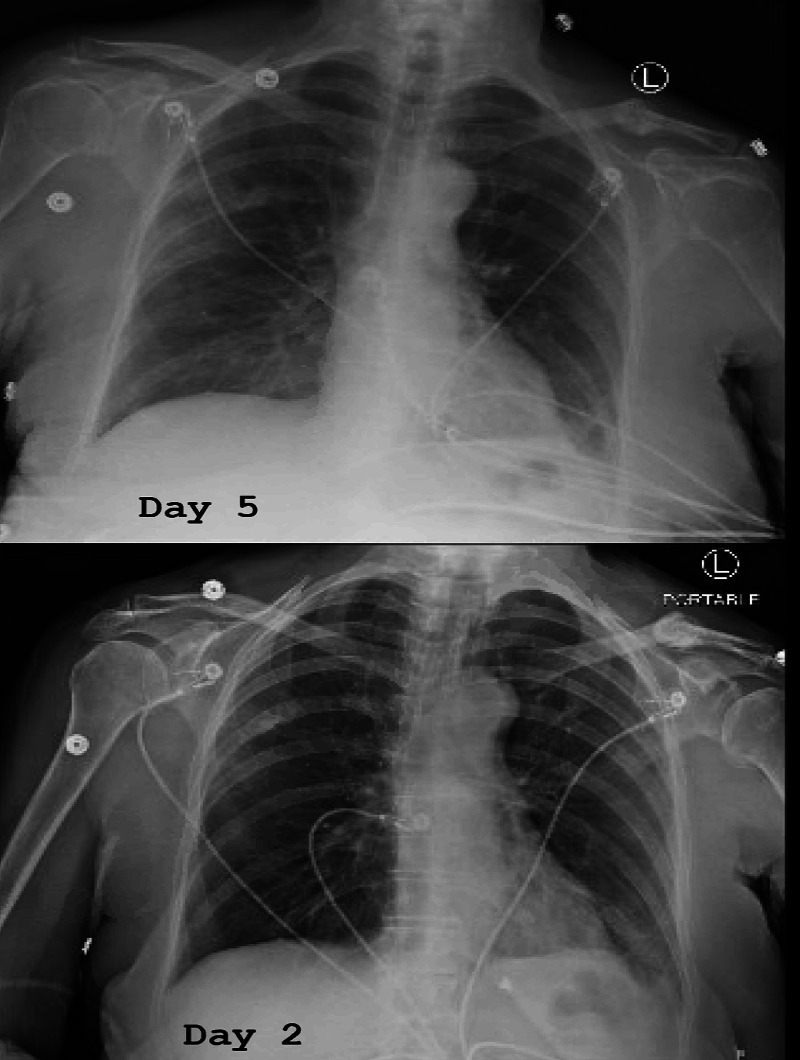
Portable CXR of the patient on day 2 and day 5 of admission CXR - chest X-ray
